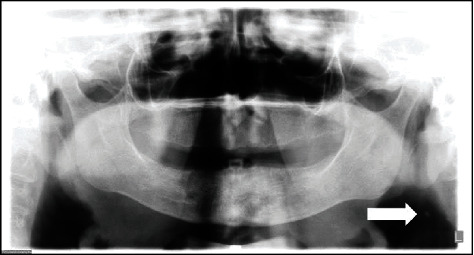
Panoramic radiograph showing the presence of radiopaque mass circumscribed on the left side of the cervical region (arrow).
